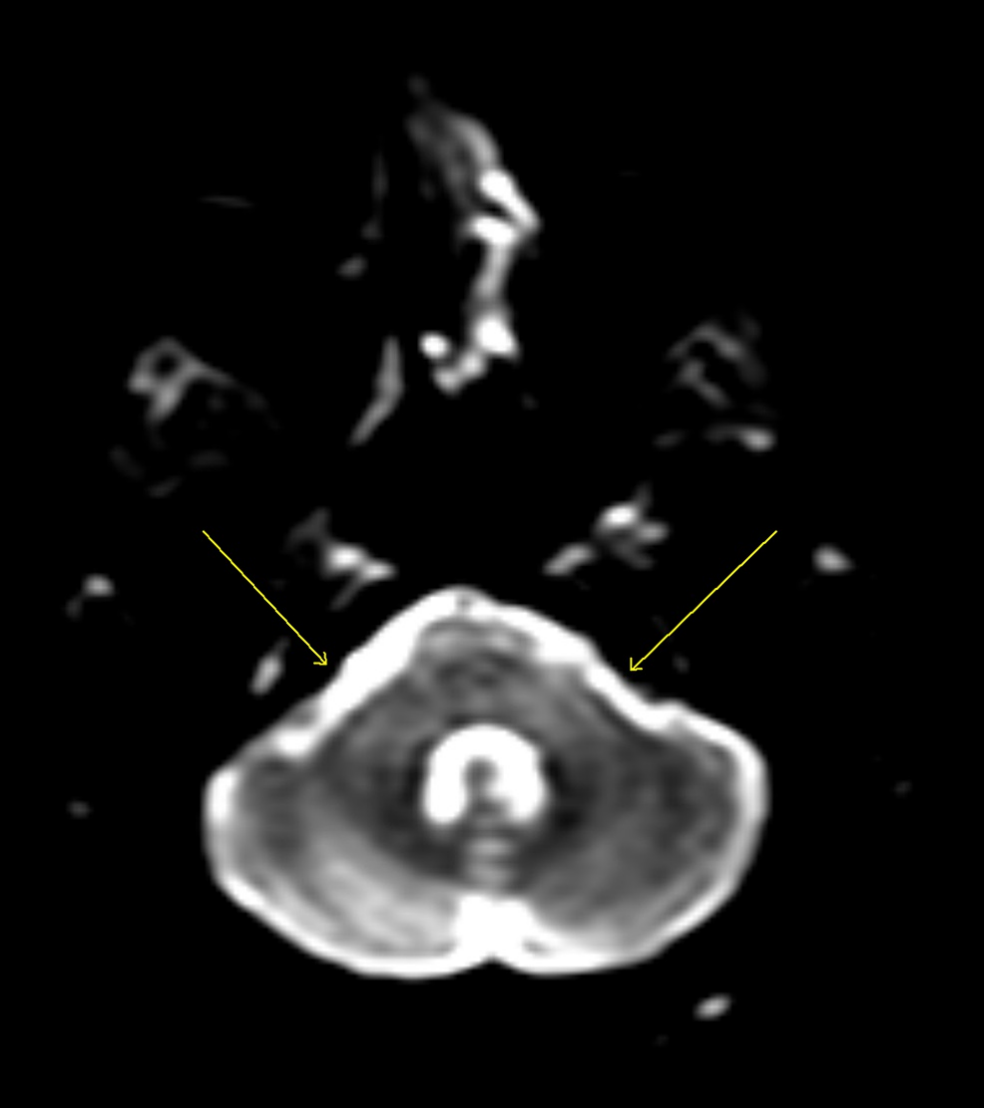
Diffusion-weighted MRI when the patient presented. Arrows show both brachium (left>right) of pons with extensive hyperintense T2-signal abnormality (also present over pericallosal and periventricular white matter suggesting demyelination).
